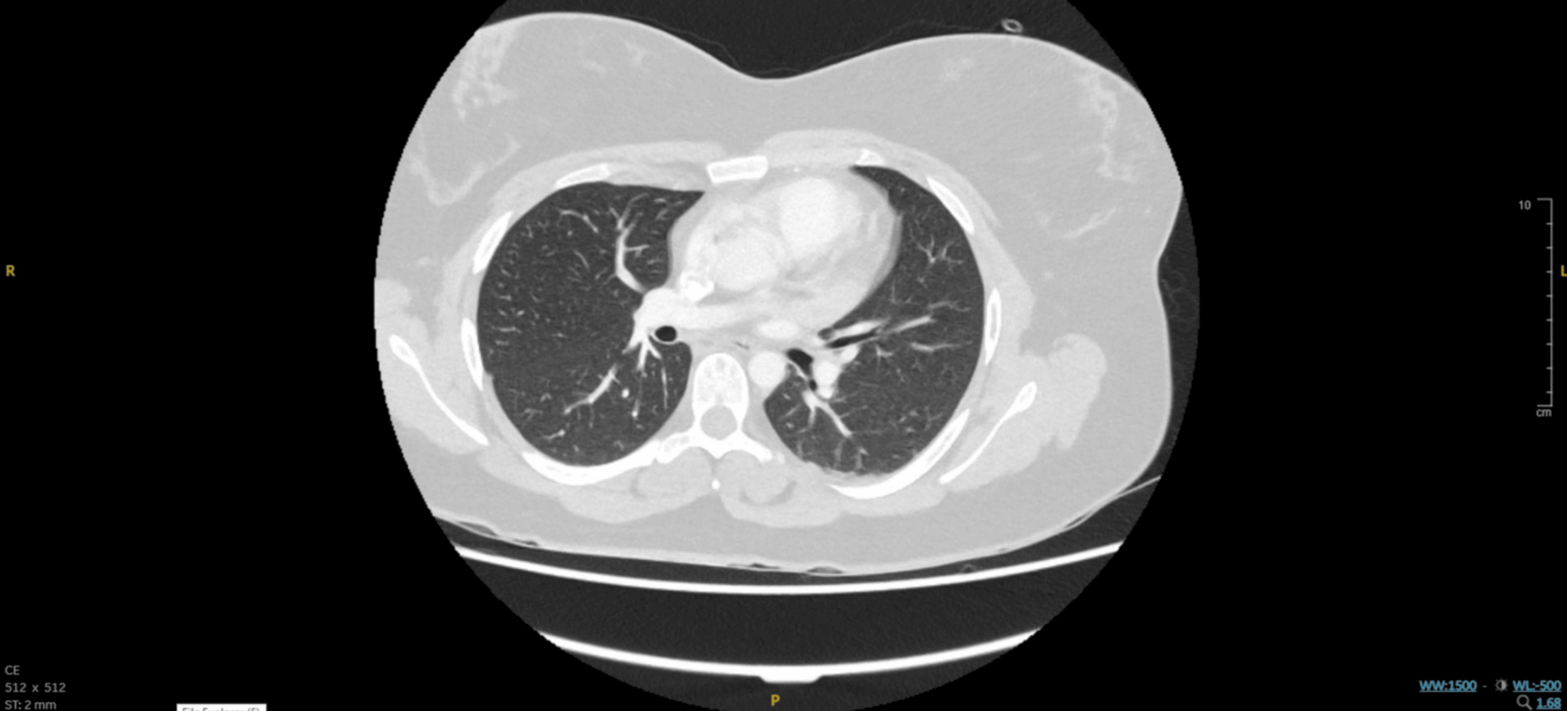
Normal contrast-enhanced CT of the pulmonary arteries (lung window)
